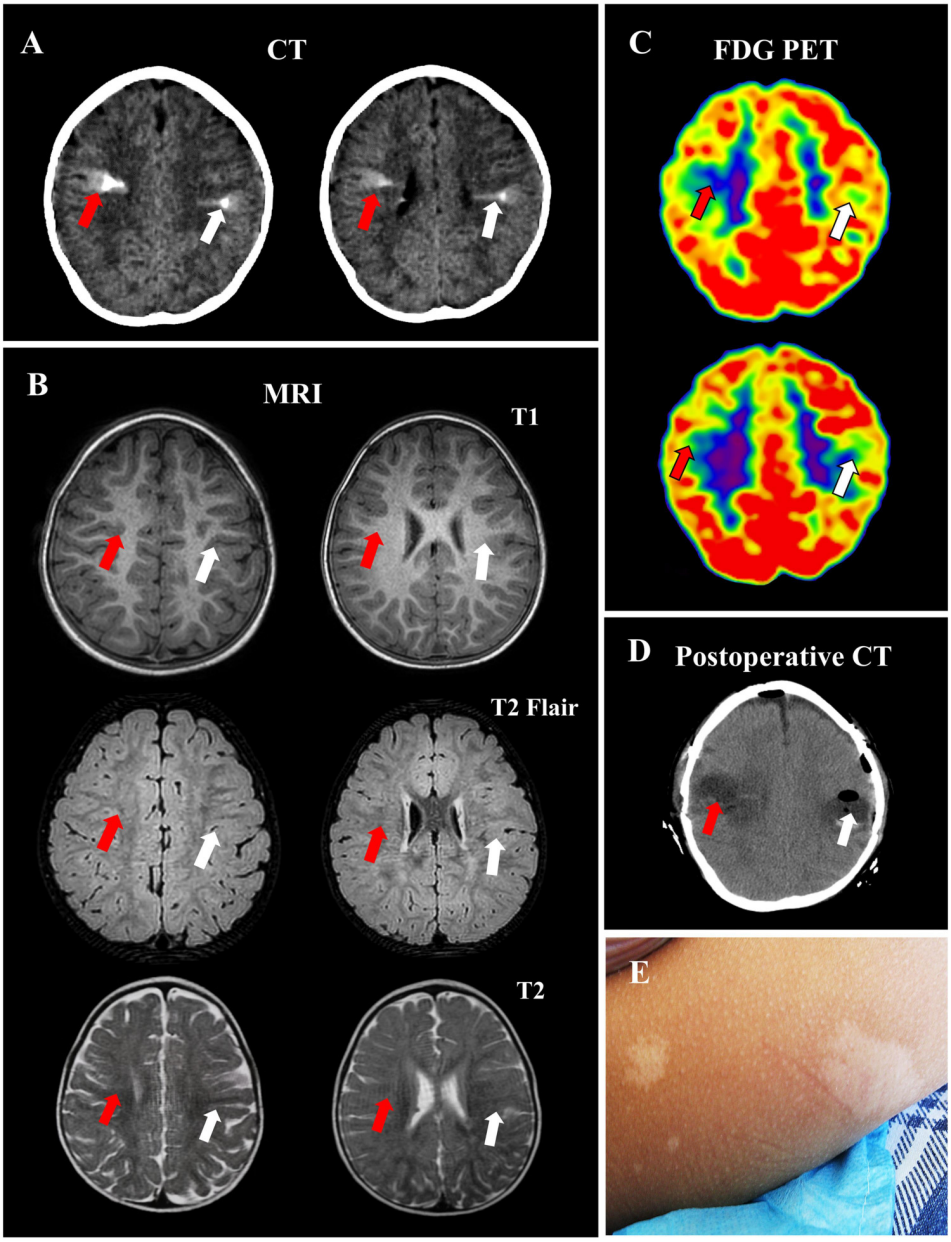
CT calcified radial lesion resembling TMS in the patient with TSC. Preoperative evaluation revealed high-density goblet-like CT lesions resembling TMS (arrows) (A) No significant hyperintensities were observed on T1, T2 Flair and T2 images from MRI (arrows) (B) Hypointense lesions were identified on FDG PET images (arrows) (C) Postoperative image shows the CT calcified radial cortical tuber were resected (D) Hypopigmentation was noted on the patient's skin (E) TMS, transmantle sign; TSC, tuberous sclerosis complex; FLAIR, fluid-attenuated inversion recovery; MRI, magnetic resonance imaging.
